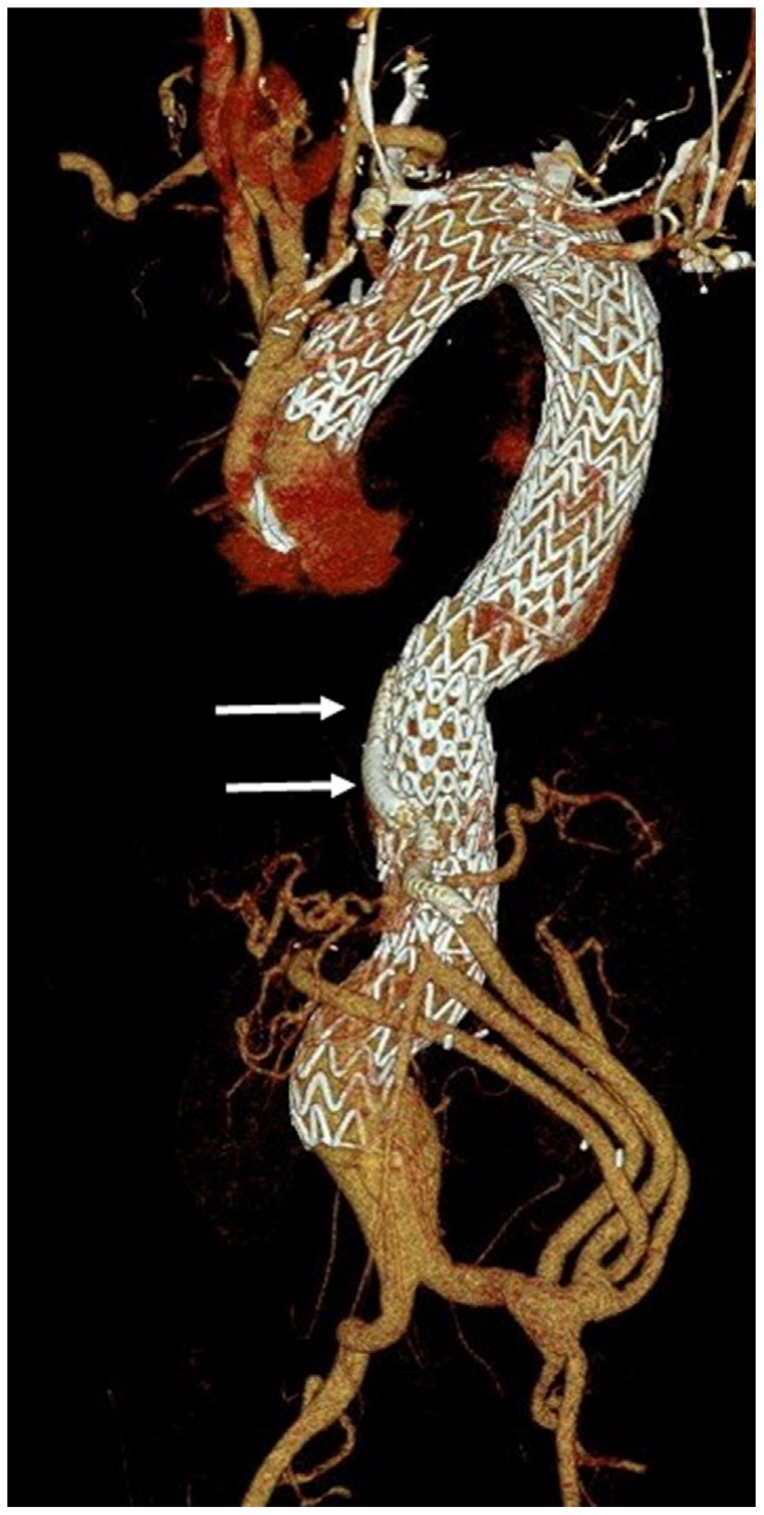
Volume-rendered reconstruction of a postoperative computed tomography (CT) arteriogram. Note the perfusion branch is occluded (*arrows*).
